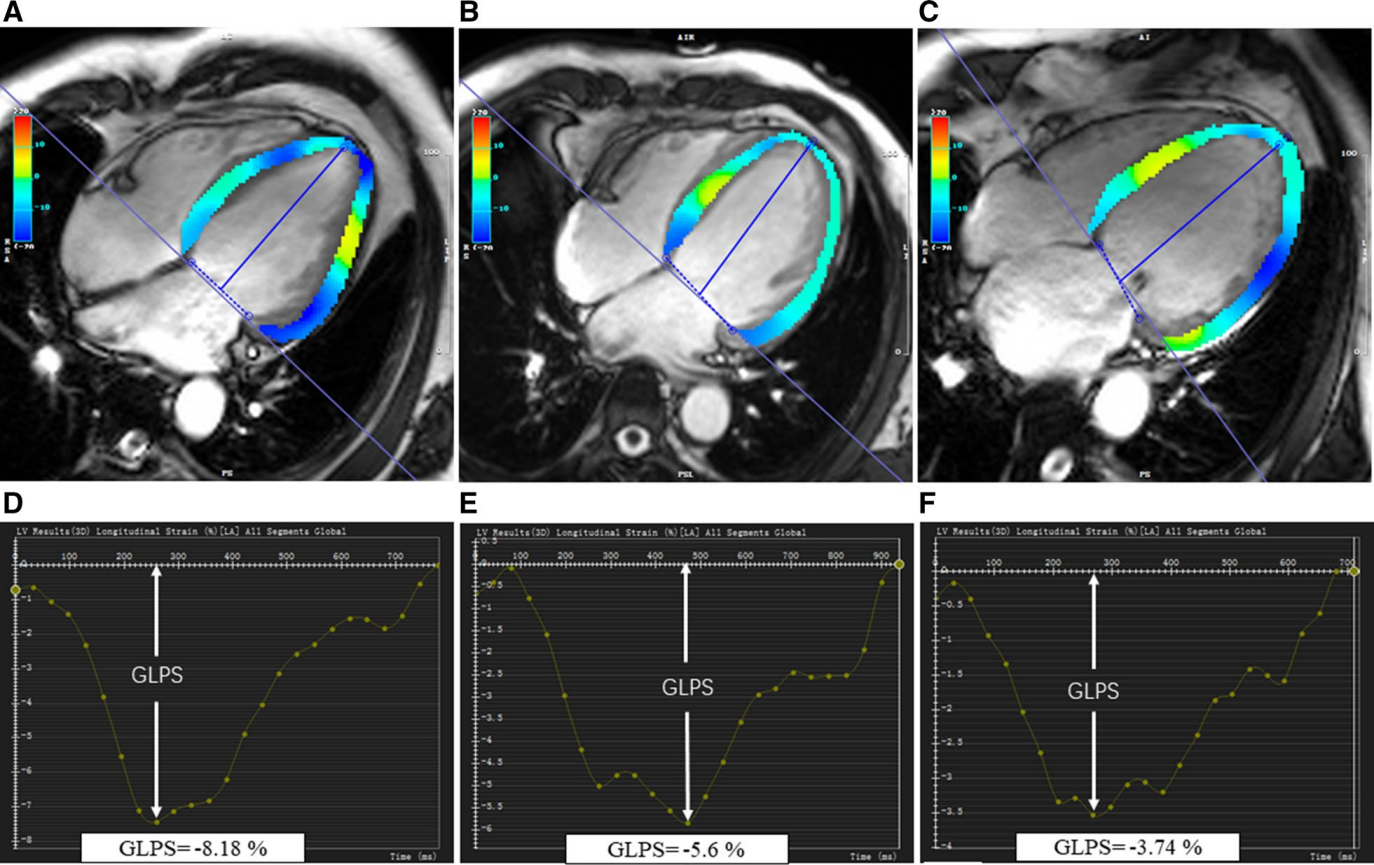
Representative cardiac MRI left ventricle pseudocolor images of long-axis four-chamber cine images at the end-systole and cardiac MRI derived global longitudinal peak strain curves of a HFrEF control patient (A, D), a hypertensive HFrEF patient (B, E), and a hypertensive HFrEF patient comorbid with diabetes mellitus (C, F)
